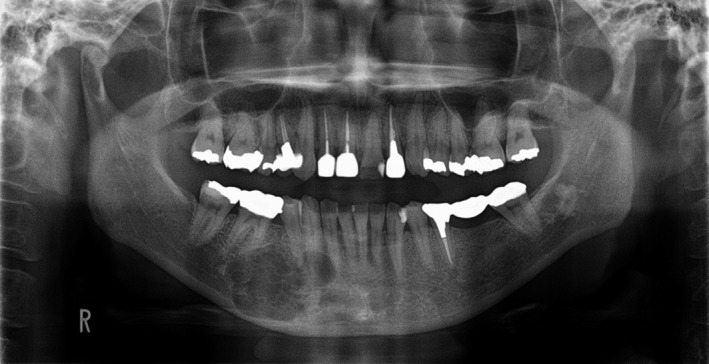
Orthopanoramic radiograph obtained during the patient's first visit. A multilocular radiolucency is visible in the right side of the mandible
